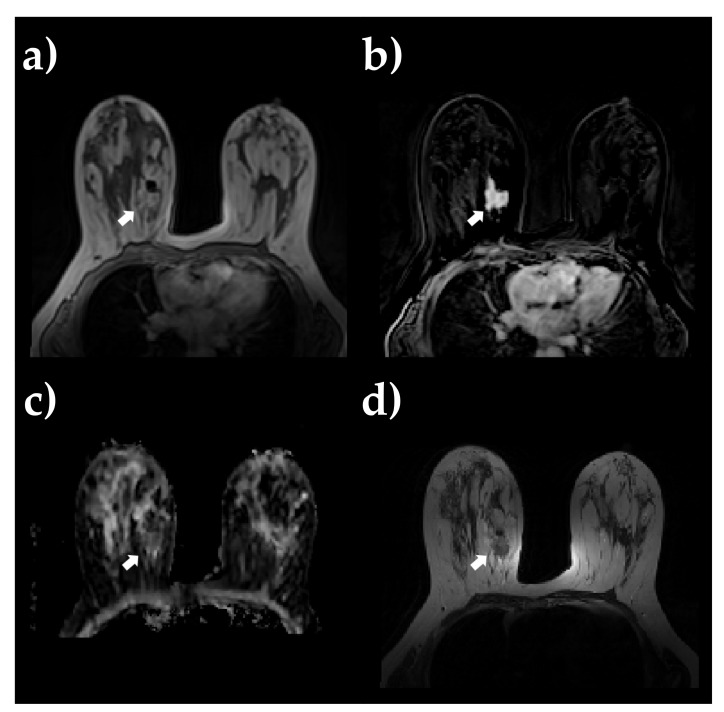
Example of primary BC lesion shown on a pretreatment breast MRI: (a) post-contrast T1 images, (b) DCE-MRI subtraction images with the highest mean signal intensity within the ROI, (c) ADC map, and (d) T2 images.
